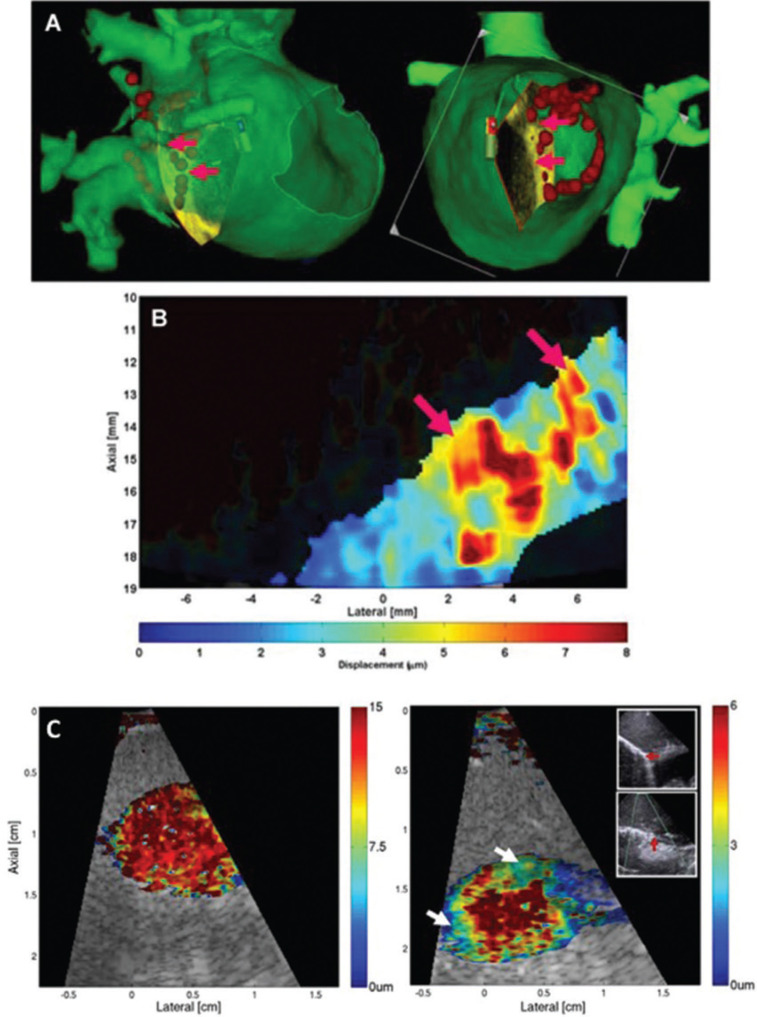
Acoustic radiation force impulse elastography (ARFIE) reveals regions of incomplete ablation. (a) Two views of a segmented and registered computed tomography scan. The intracardiac echocardiography imaging plane is seen projected on the sepal margin of the right inferior pulmonary vein (PV) antrum and yields the image in (b). Radiofrequency ablation (RFA) tags are marked in red, and the red arrows designate regions with intact conduction (noted on lasso recordings). (b) The ARFIE image demonstrating stiff ablated areas (blue) and soft un-ablated areas (red) corresponding with sites of incomplete ablation and PV connection. (c) A left atrial ARFIE image of the ligament of Marshall before ablation; the tissue was uniformly soft (appearing red) consistent with unablated tissue (left). After two RF pulses (see inset), the ARFIE image shows two discrete lesions that are incomplete (arrows). Adapted from: Bahnson TD, Eyerly SA, Hollender PJ, et al. Feasibility of near real-time lesion assessment during radiofrequency catheter ablation in humans using acoustic radiation force impulse imaging. *J Cardiovasc Electrophysiol.* 2014;25(12):1275–1283.
